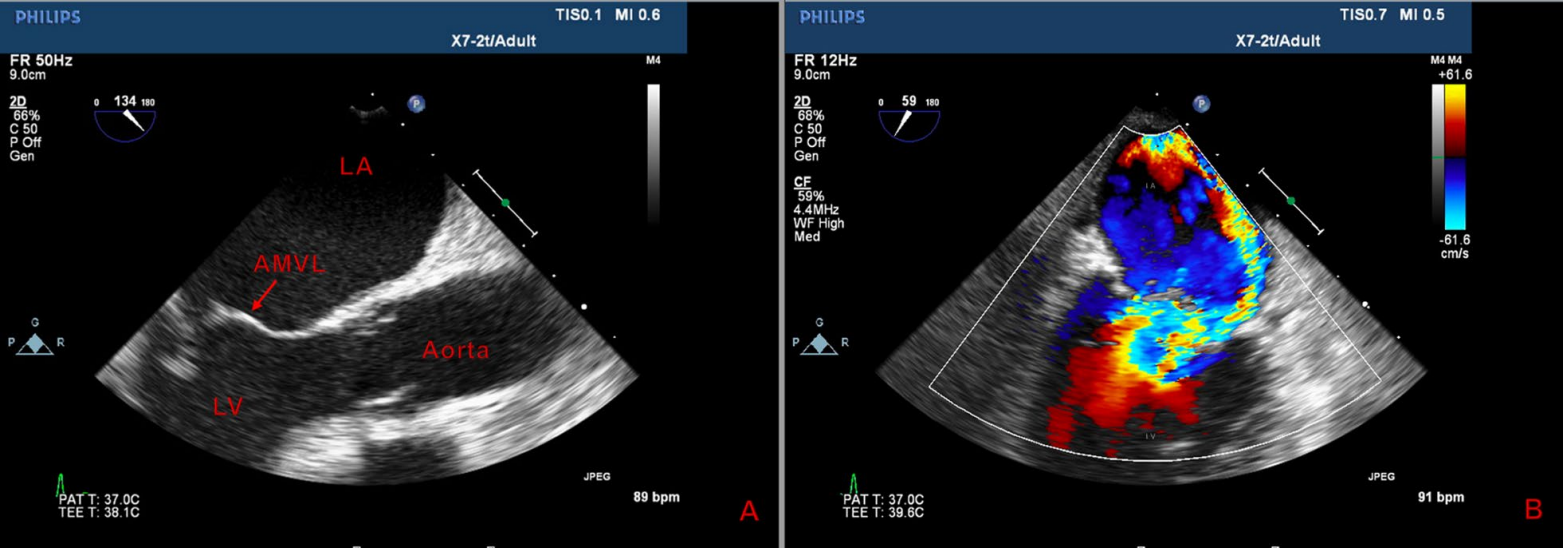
Mitral valve imaging. Flail anterior mitral valve leaflet is seen on transesophageal echocardiogram (A) with evidence of a posteriorly directed regurgitant jet (blue) on transthoracic echocardiogram (B)
